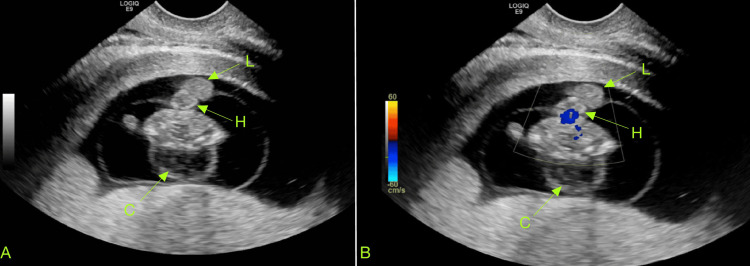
Transverse ultrasound view showing the fetal heart and part of the herniated liver outside the fetus. Image A provides a standard view for comparison, while image B is a Doppler ultrasound demonstrating blood flow through the heart C: cystic hygroma, H: heart, L: liver
